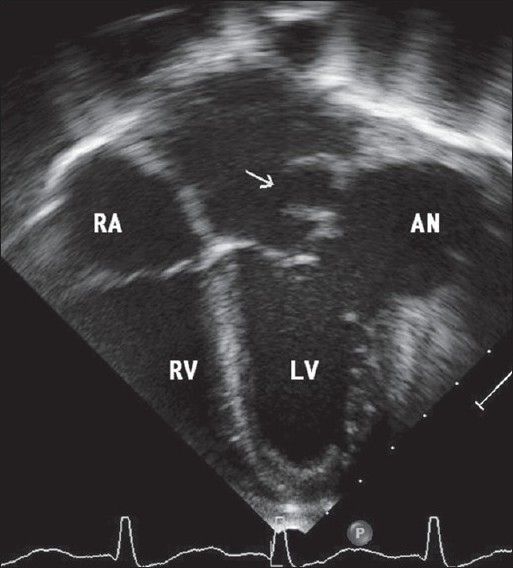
Two dimensional echocardiogram in apical four chamber view shows a large submitral aneurysm adjecant to the lateral papillary muscle of the mitral valve. It extends superiorly and is seen to rupture into left atrium (arrow)
